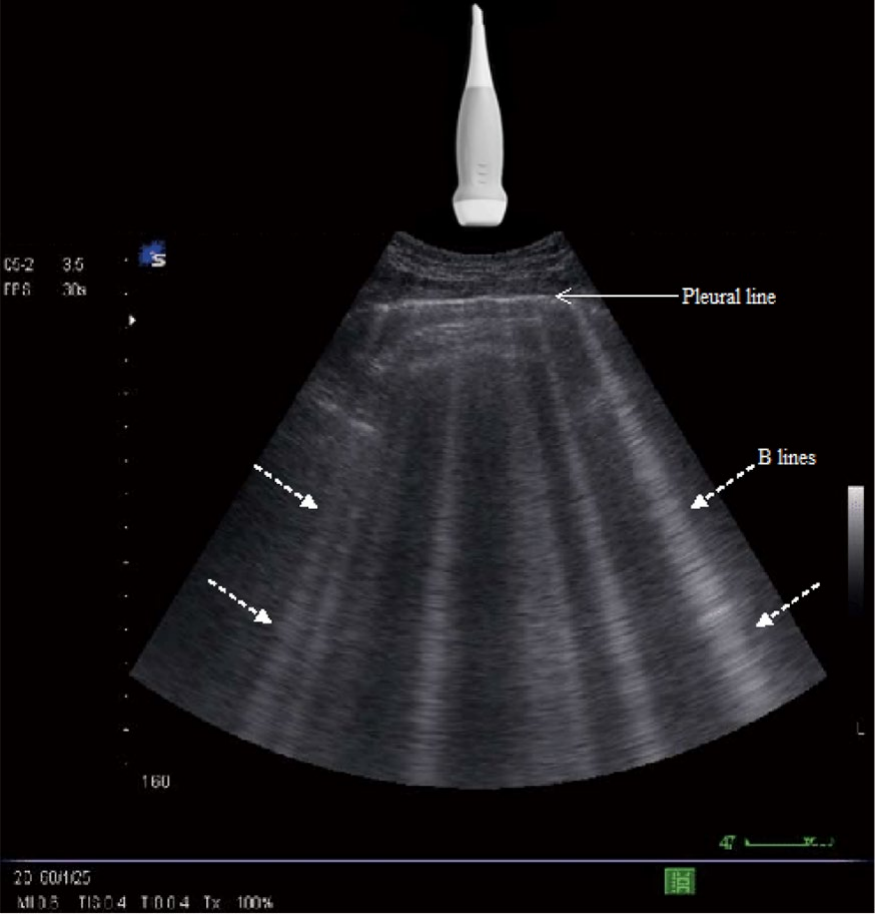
B-lines represented by vertical hyperechoic images starting from the pleural line and extending to the whole ultrasound field (discontinuous arrows)
